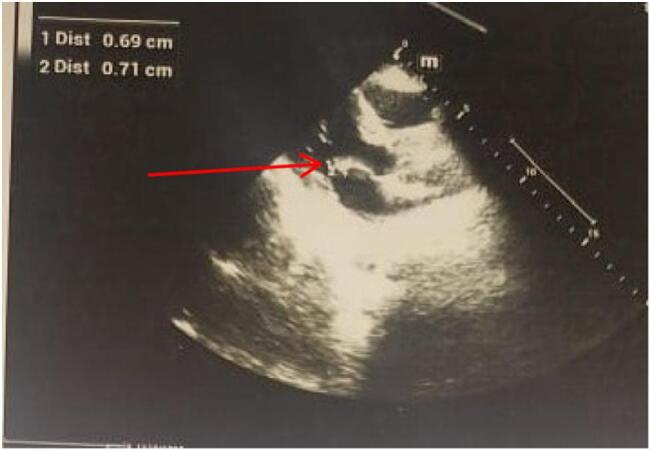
Echocardiography showed thickened anterior mitral valve leaflet with no evidence of vegetation (red arrow).
